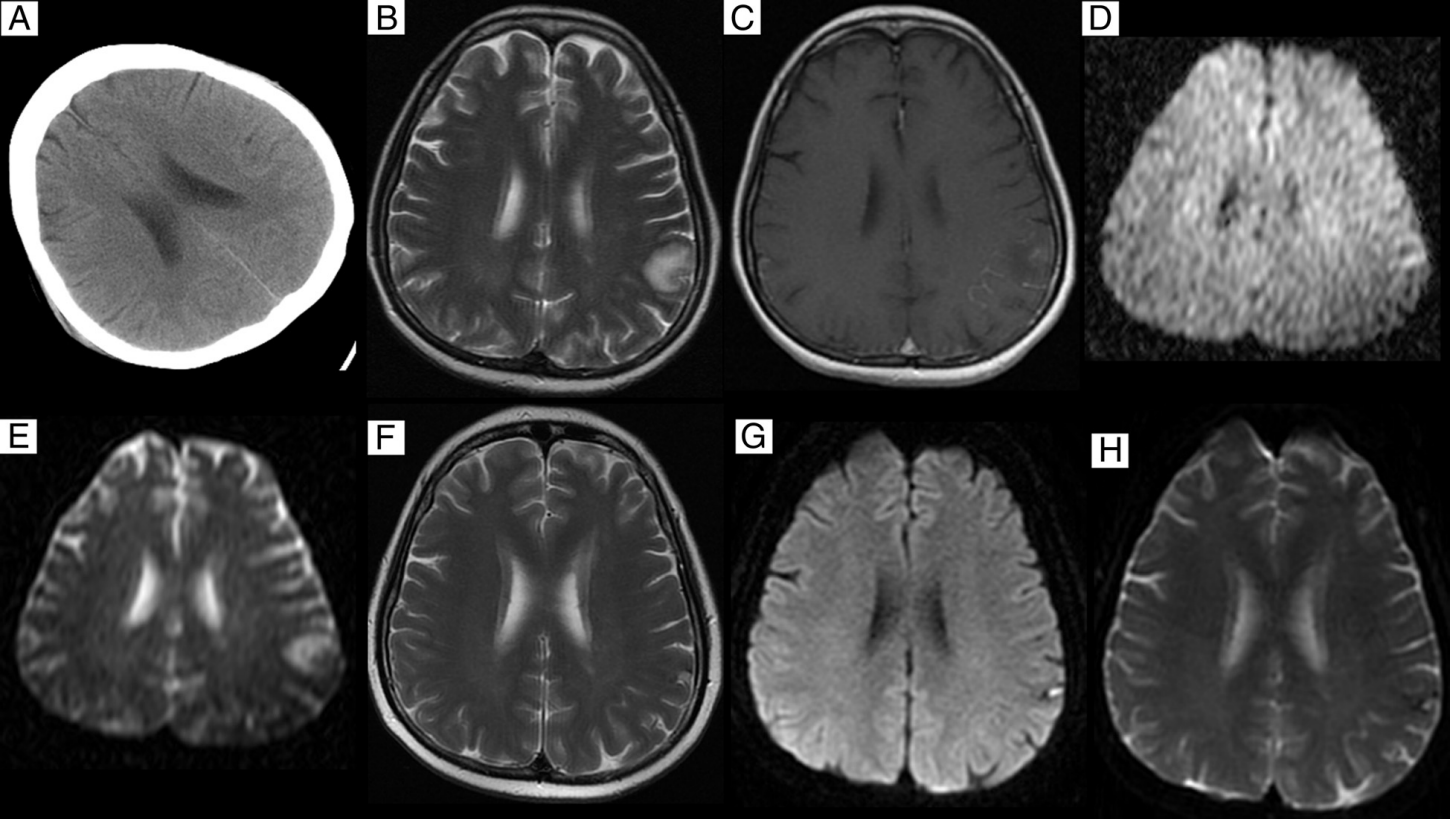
Brain CT and MRI on admission and after treatment in a 26 year-old SLE patient who presented with seizure. CT (A) shows normal but T2-weighted image (B) shows high signal lesions in subcortical left centroparietal white matter. On contrast-enhanced T1-weighted sequence (C), there is minimal enhancement at the periphery. Diffusion-weighted image (D) shows high-signal intensity area in corresponding lesion areas. Corresponding ADC map (E) reveals high signal intensity area. The abnormal lesion completely diminished in T2-weighted image (F) after 8 months of steroid therapy. DWI (G) shows high signal lesions and ADC (H) reveals lesion low intensity.
